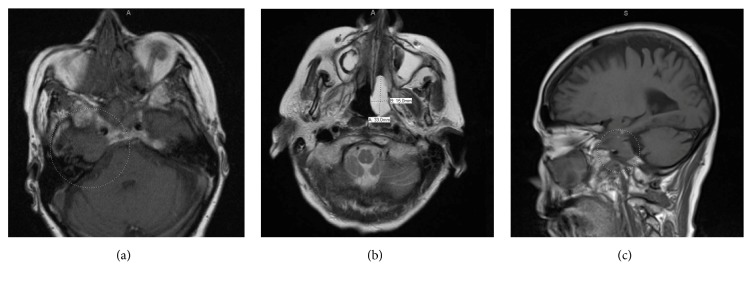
MRI brain: three abnormal bone marrow lesions (left clivus, right clivus, and occipital bone). The right clivus lesion appears to extend to the dura along the medial aspect of the middle cranial fossa which is likely the cause of cranial nerve sixth palsy. (a) Axial section at the level of the pons. (b) Axial section at the level of the medulla. (c) Sagittal section.
